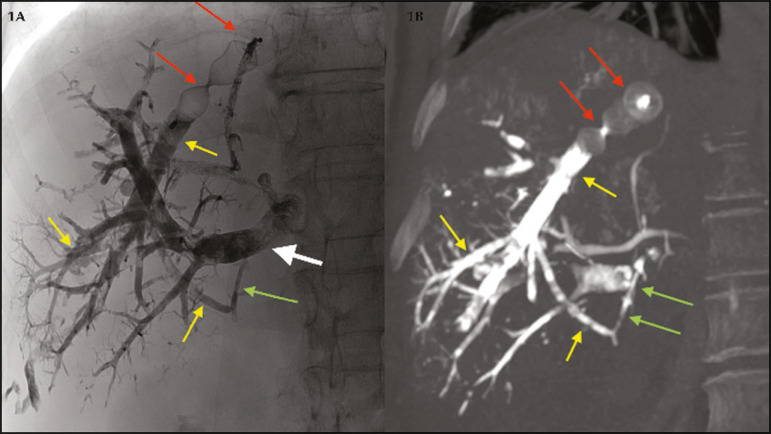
Fluoroscopic image (1A) obtained immediately after LVD and contrastenhanced coronal CT (1B) obtained 14 days after LVD. Note the vascular plug (red arrows) placed in the right hepatic vein for proximal embolization and NBCA plus lipiodol occluding the distal branches (yellow arrows). Note also NBCA plus lipiodol occluding a venovenous collateral (green arrows) and right portal vein embolization with NBCA plus lipiodol (white arrow).
